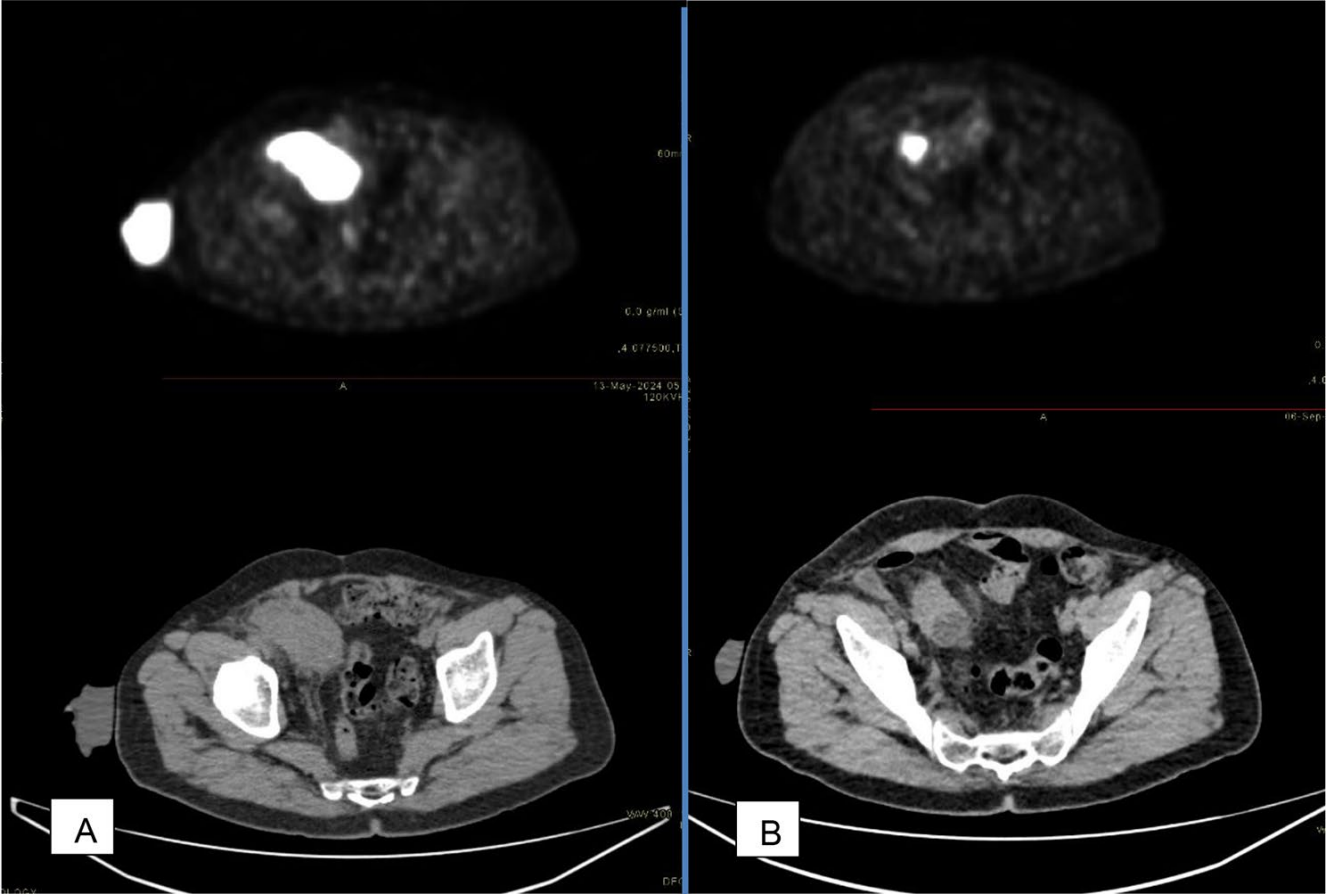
(A) FDG PET/CT scan before initiation of chemotherapy (B) Post-chemotherapy scan
